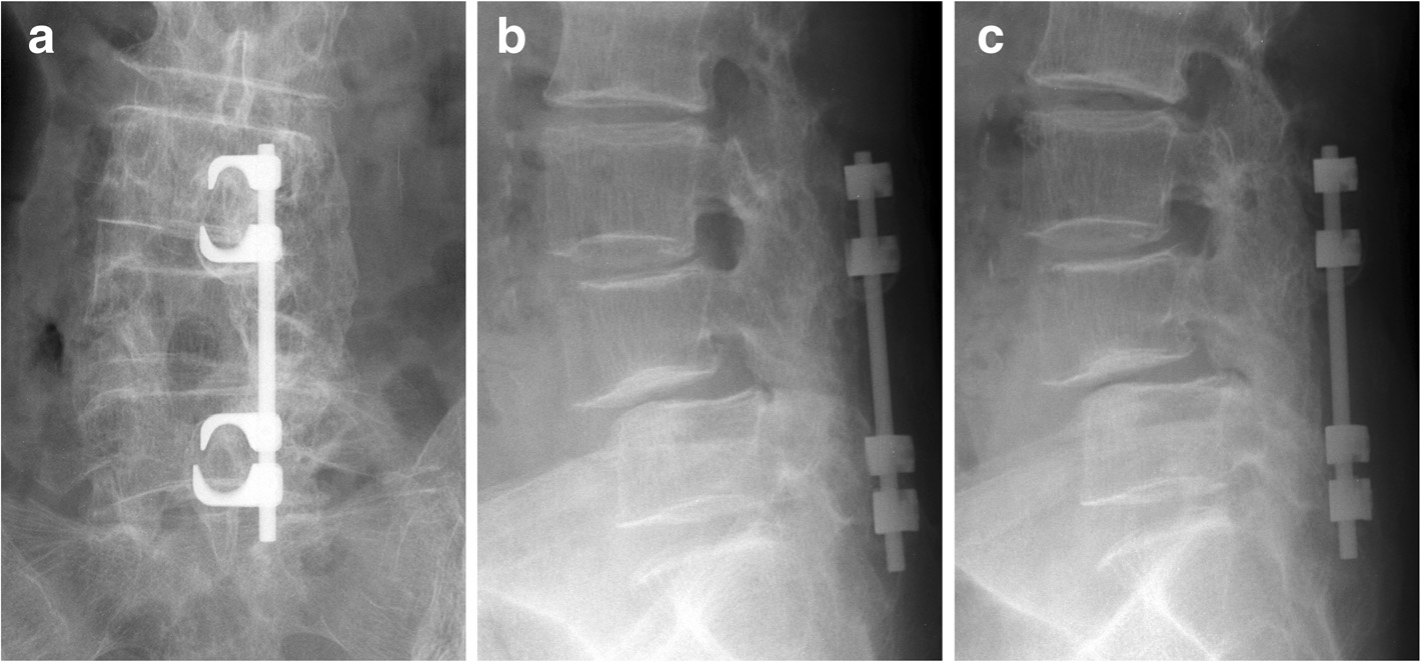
Preoperative X-rays 5 years after surgery of an 80-year-old female. The X-rays show a good fusion mass and no evidence of adjacent segment degeneration. (a) Anteroposterior view. (b) Lateral view of anteflexion. (c) Lateral view of retroflexion.
